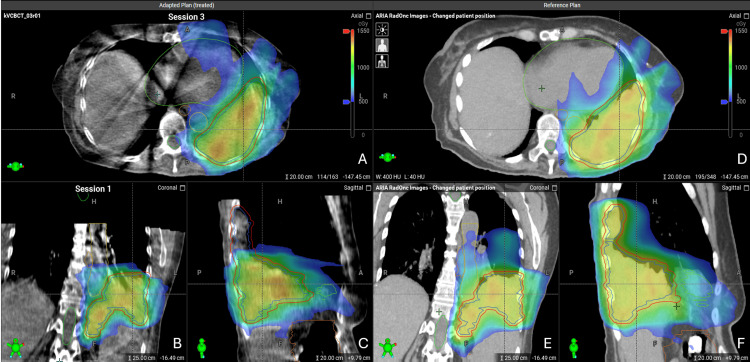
Third Fraction of Online Adaptive Radiotherapy to a Bulky Thoracic and Abdominal Mass Cone-beam computed tomography (CBCT) scan from the third fraction of adaptive stereotactic body radiotherapy (SBRT) compared to reference plan on planning CT (pCT) with dose colorwash overlay. The cumulative point-dose maximum delivered to the heart across the treatments approached the 29 Gray (Gy) maximum dose constraint. Gross tumor volume (GTV) and planning target volume (PTV) coverages were compromised at the discretion of the treating radiation oncologist to protect the heart based on clinical judgment. The axial-adapted CBCT is shown in (A). The superior portion of the mass was blocked to decrease dose to OARs, best seen on coronal (B) and sagittal (C) views. The associated reference plan is shown for comparison with axial (D), coronal (E), and sagittal (F) views. Contours from the adapted plan are displayed on both CBCT and pCT showing GTV (blue), PTV (red), great vessels (gold), colon (orange), esophagus (brown), heart (light green), and spinal cord (dark green).
